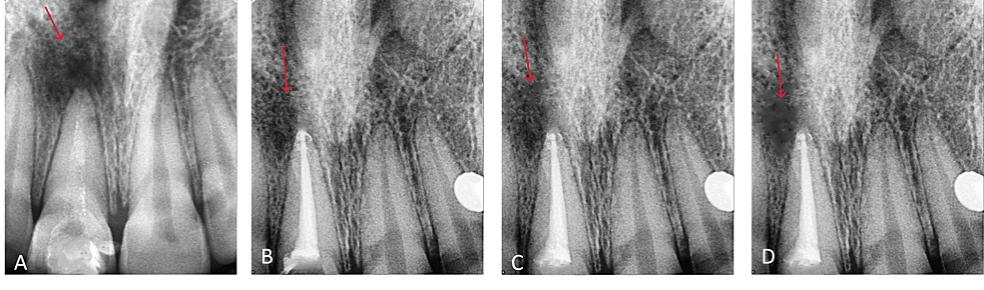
(A) Pre-operative intra-oral periapical radiograph with tooth number 11; (B) post-obturation radiograph with tooth number 11; (C) six-month follow-up radiograph; (D) 12-month follow-up radiograph.
